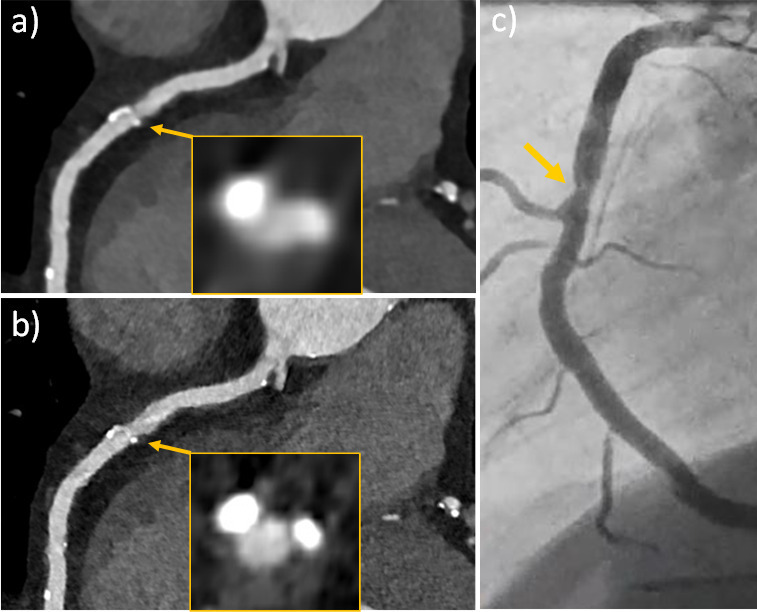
Ultra-high-resolution cCTA of a 62-year-old male patient on a clinical PCD-CT, prior to transcatheter aortic valve replacement. Heart rate during data acquisition: 74 bpm. (a) Standard reference reconstruction (0.6 mm slice, Bv40, QIR4). A moderate stenosis (60% in diameter) is seen in the proximal RCA (arrow). (b) UHR reconstruction (0.2 mm slice, Bv60, QIR4). Note the reduced calcium blooming, leading to a re-classification to a mild stenosis (38% in diameter). (c) Invasive catheter coronary angiography confirmed a mild stenosis in the proximal RCA (arrow). cCTA, coronary CT angiography; PCD-CT, photon-counting detector CT.
